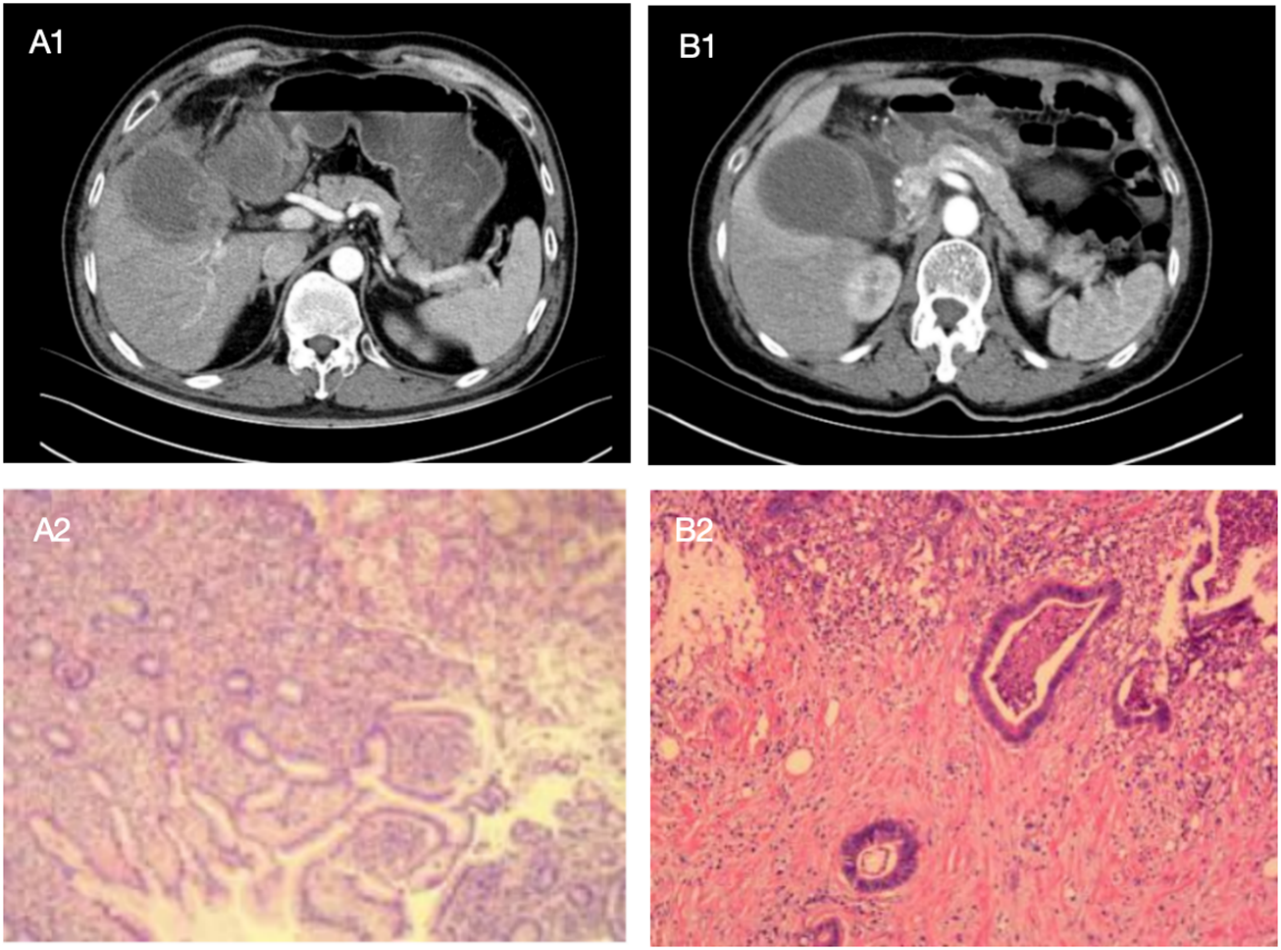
The following are two cases in which the model was applied to intraoperative decision making, with preoperative enhanced CT images of patients and postoperative pathological sections as above. Patient A showed a high-density mass with blurred margins in the arterial phase of enhanced CT. The mass was located in the neck of the gallbladder. The patient was a 52-year-old man with Murphy's sign, N 7.4*10^9/L, GGT 149U/L, and CEA 0.8ug/L. Comprehensive preoperative imaging diagnosis (A1) was considered suspicious of gallbladder cancer, but its probability of predicting gallbladder cancer was 0.239. In this case, the boundary and anatomical structure of the liver of the gallbladder were blurred during the operation, and the frozen section pathology showed no abnormality after complete resection of the gallbladder. The surgeon chose simple cholecystectomy based on the conjecture of the model, and no "gallbladder cancer" was found in postoperative routine pathology (A2). Patient B showed a high-density mass with blurred margins in the arterial phase of enhanced CT. The mass was located in the neck of the gallbladder. The patient was a 68-year-old female with negative Murphy's sign, N 4.35*10^9/L, GGT 15U/L, and CEA 2.3ug/L. Comprehensive preoperative imaging diagnosis (B1) considered benign gallbladder disease, but its probability of predicting gallbladder cancer was 0.880. In this case, no gallbladder cancer was found by repeated frozen pathology during operation, but radical resection of gallbladder cancer was still performed after the surgeon conjecturing according to the model, and postoperative routine pathology (B2) showed "gallbladder cancer".
